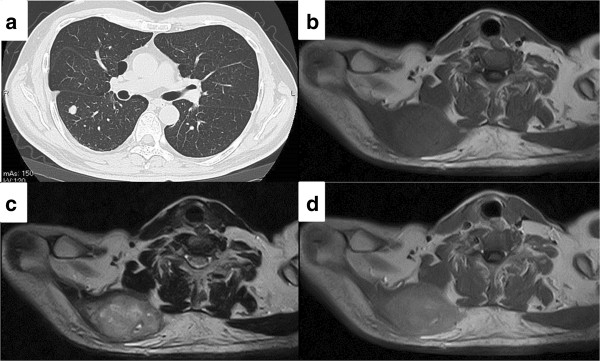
CT and MRI imaging of metastatic osteosarcoma. (a) Computational tomography (CT) imaging of lung metastasis in the early course of the disease. Multiple metastases are seen in both lungs. Axial (b) T1-weighted and (c) T2-weighted magnetic resonance imaging (MRI) scan shows a heterogeneous, ill-defined mass measuring 73 × 45 × 60 mm in the right trapezius muscle. (d) Gadolinium-enhanced T1-weighted image shows a circumscribed signal abnormality in the right trapezius muscle that was moderately enhanced.
